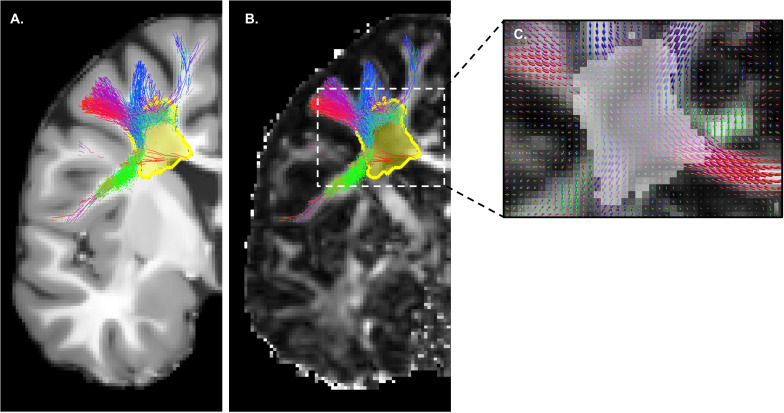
Tracking through white matter lesions. Manual and automatic segmentations are fused to produce the “lesion mask” (in yellow), covering all white matter lesions visible on the T2-FLAIR and T1-weighted (A) MRI images. Probabilistic tractography based on fODF peaks (C) is then executed and tracking is allowed through lesions, as shown on the FA map (B). Without this correction, no track would be generated inside the yellow mask.
